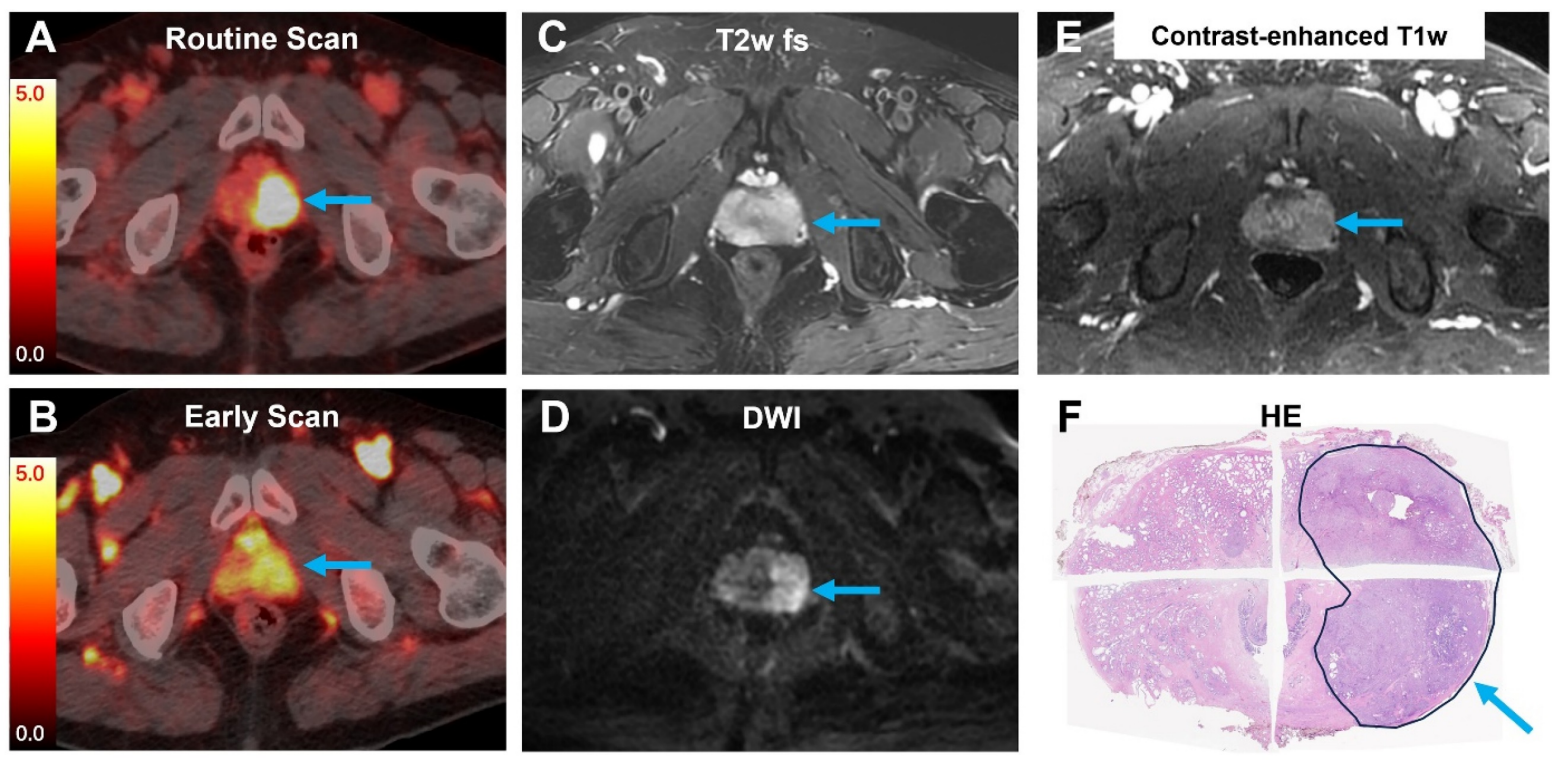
A 71-year-old man with PCa underwent pelvic MRI and [^18^F]AlF-Thretide PET/CT. His total PSA level was 21.0 ng/mL prior to the PET/CT scan. (A-B) Both the routine PET/CT scan and the early PET/CT scan showed intense [^18^F]AlF-Thretide uptake in the left lobe of the prostate (arrow, SUVmax 17.0 on the routine scan, 4.6 on the early scan). (C-E) MRI revealed a lesion with low T2 signal intensity, high DWI signal intensity, and early enhancement on the contrast-enhanced T1-weighted sequence. The lesion's margins were indistinct across all these MRI sequences. (F) The extent of the intense [^18^F]AlF-Thretide uptake was consistent with postoperative pathology results.
